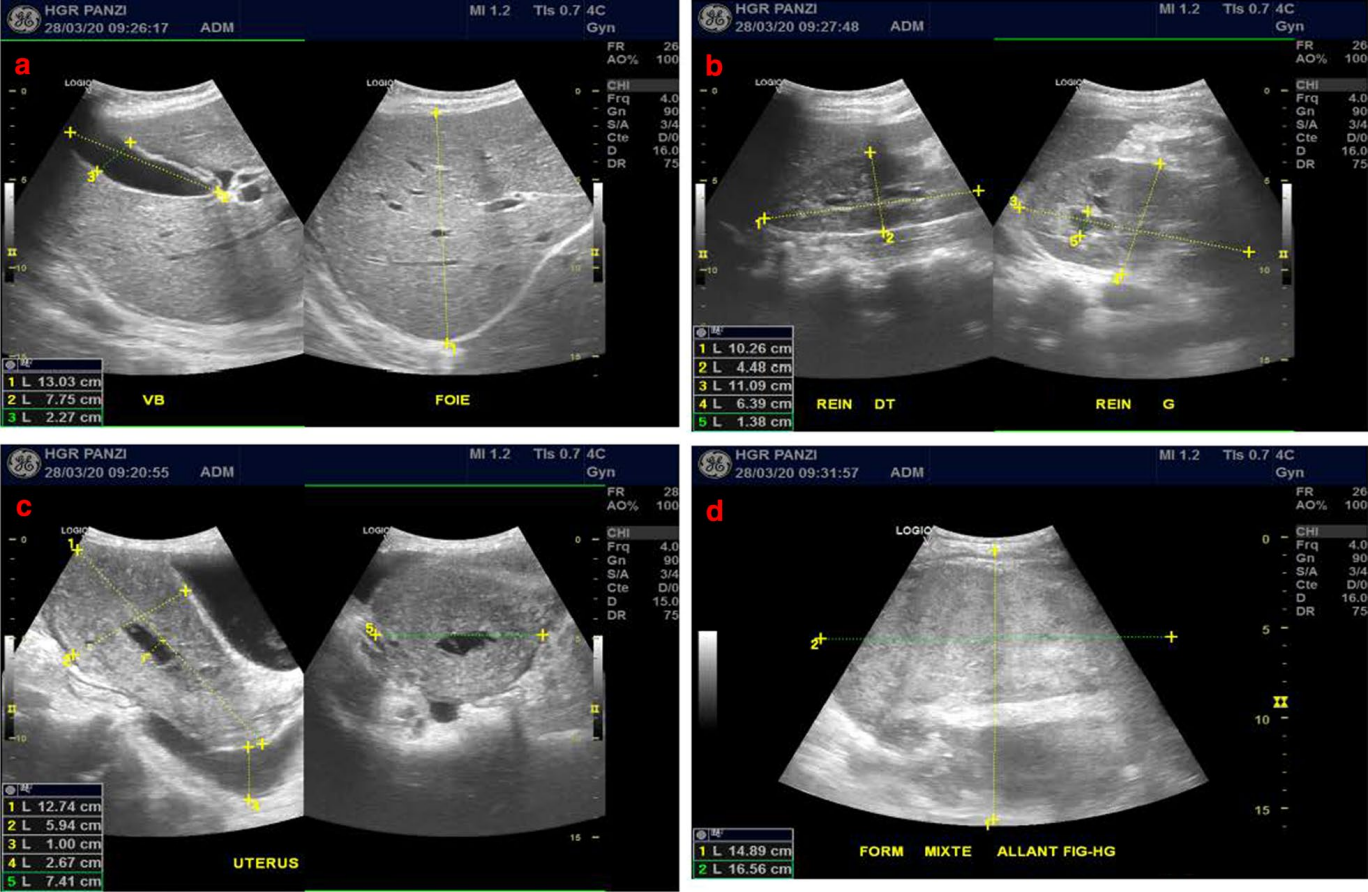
Ultrasound images showing the liver (a), kidneys (b), uterus and pelvis (c), hematoma (d)
